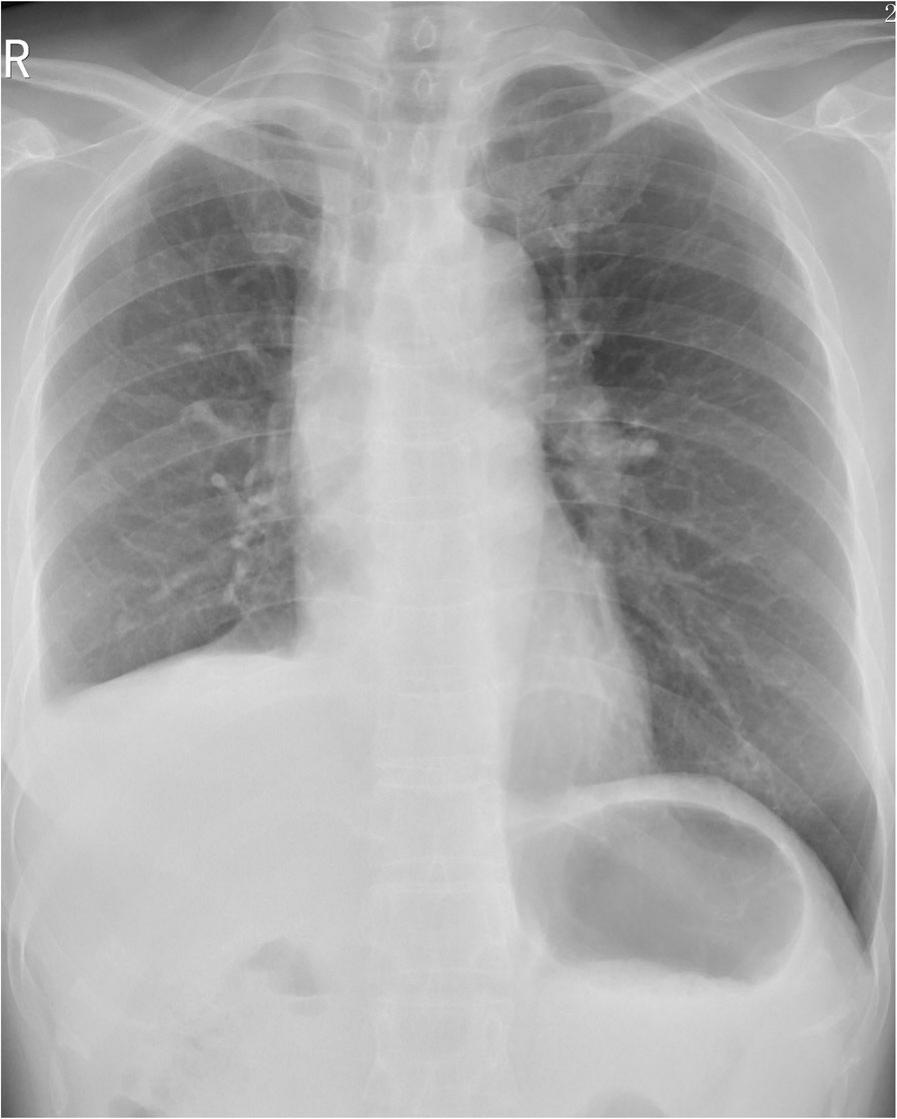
Reexamine with chest radiography half a month after discharge, no abnormality was observed
